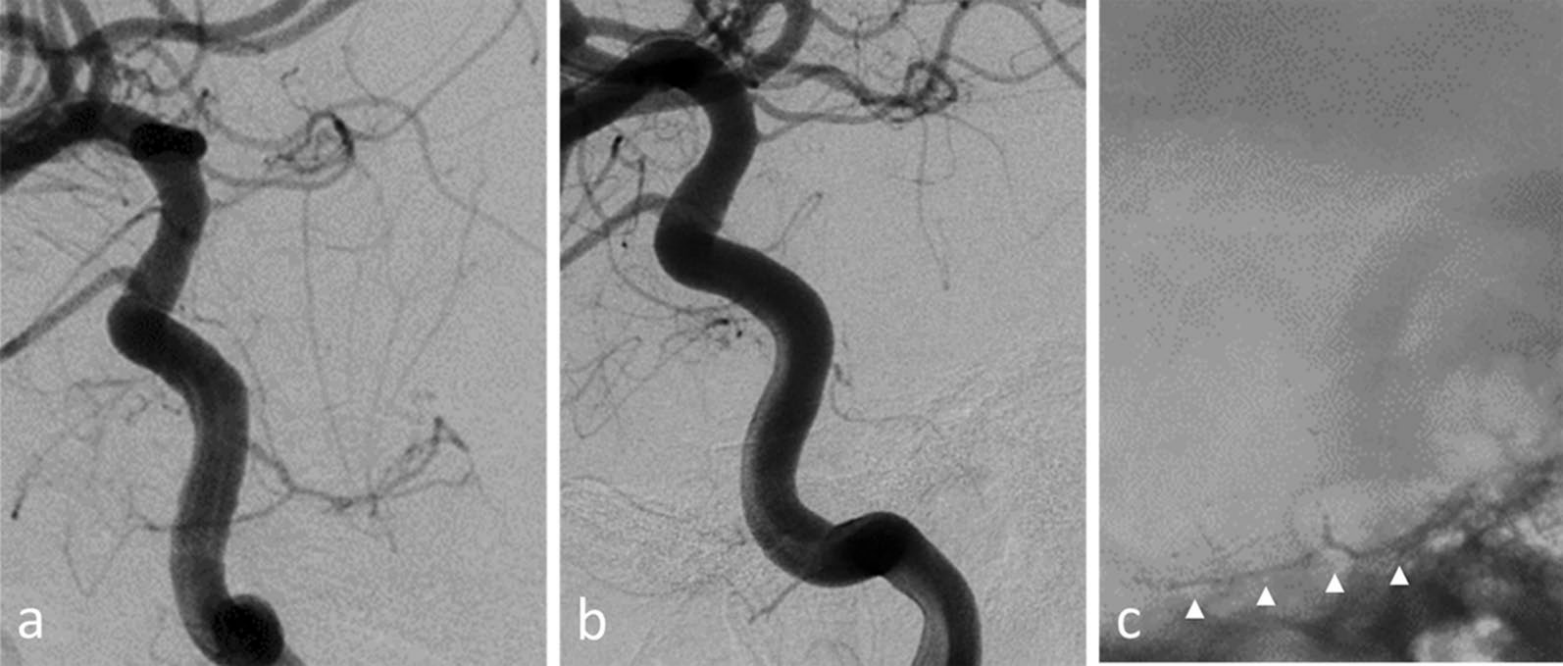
a, b Right internal carotid angiogram, oblique view, before (a) and after (b) embolization of the right meningohypophyseal trunk, showing the marked devascularization of the tumor. c Skull radiograph, oblique view, after embolization showing the cast of n-butyl cyanoacrylate (*arrowheads*)
